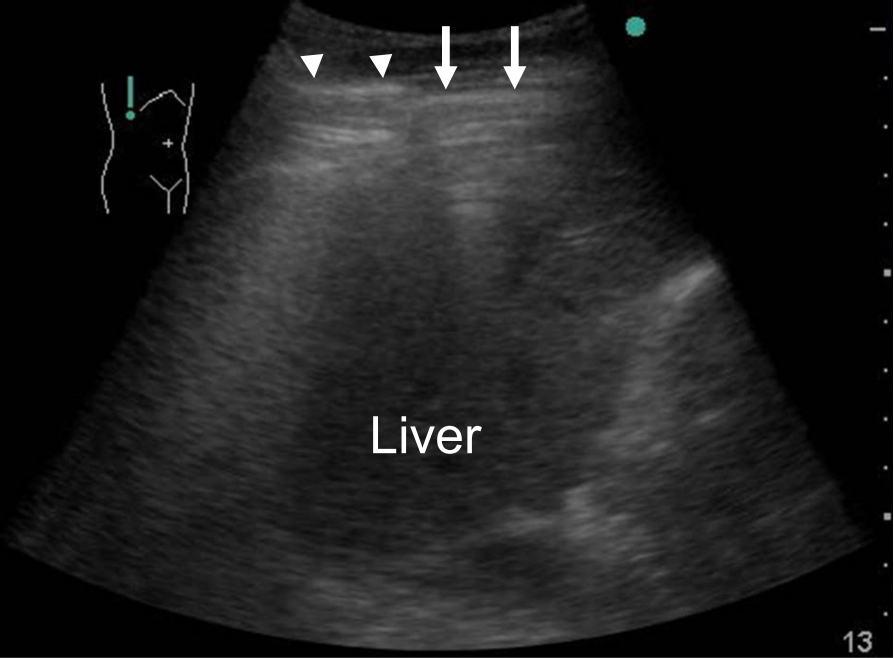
An ultrasound image in a 43-year-old man who presented with sudden onset of abdominal pain. The patient had a history of a duodenal ulcer and was aware of black stool prior to the presentation. On physical examination, he had diffuse abdominal tenderness with guarding. Bedside ultrasound was performed with the patient in the left lateral decubitus position. Reverberation artifacts on the ventral surface of the liver (*arrows*) indicated intraperitoneal free air. The artifacts were distinguished from other artifacts with respiratory movement (*arrowheads*), which originated at the lung surface
